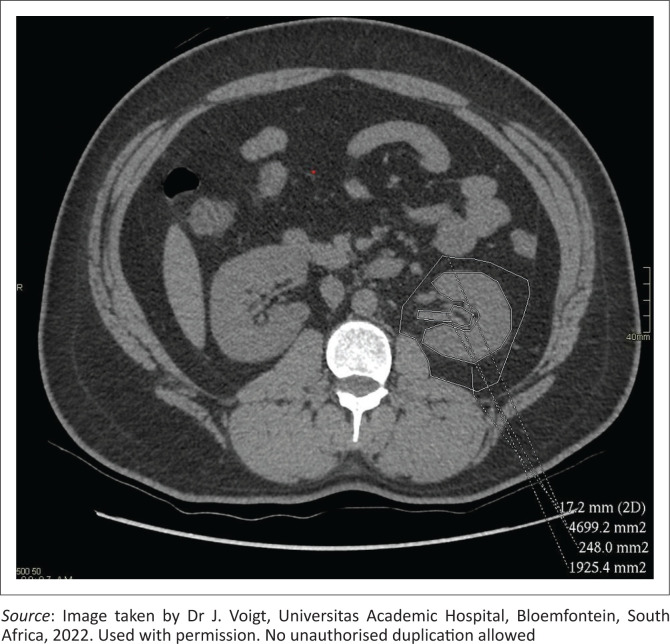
Calculating perirenal fat volume and perirenal fat thickness with manual tracing software at the level of the renal vein on non-contrasted axial computed tomography.
